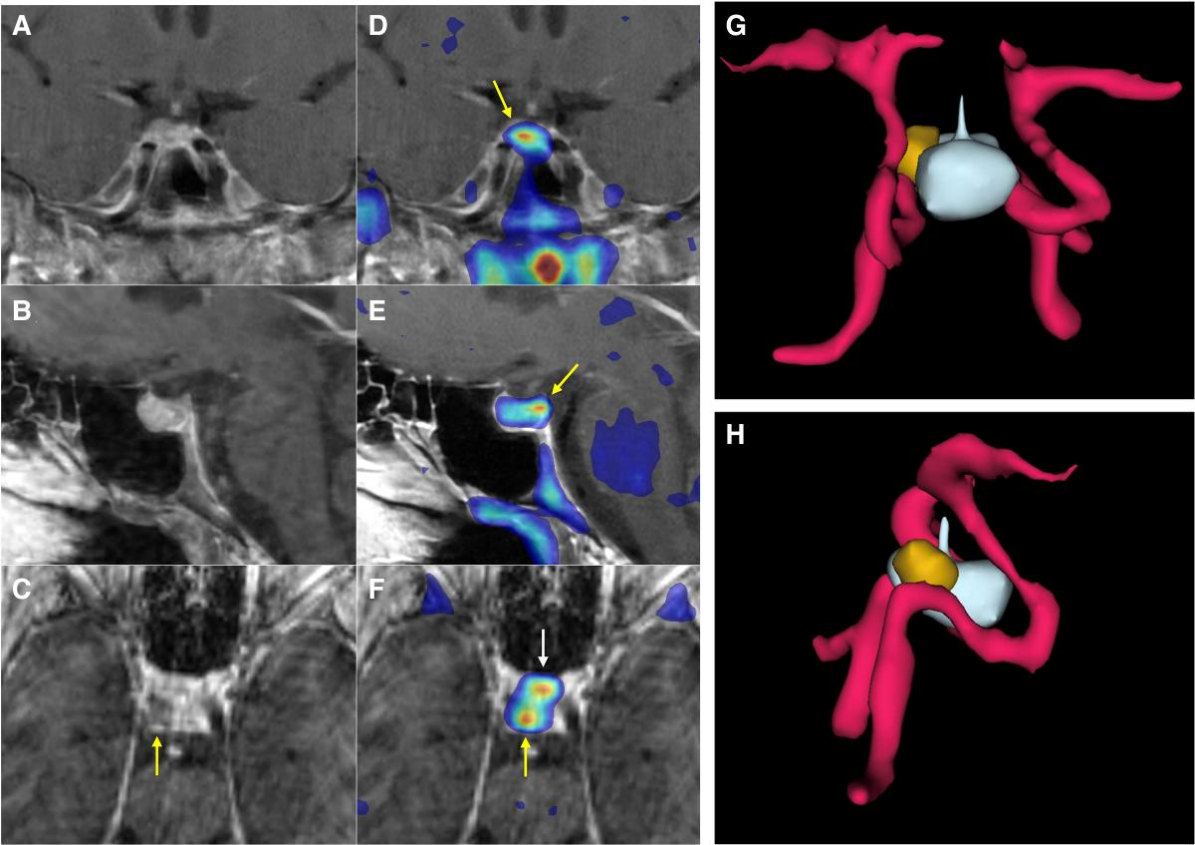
11C-Methionine PET/CT coregistered with volumetric MRI (MET-PET/MR^CR^) following treatment with osilodrostat. A subtle area of reduced gadolinium enhancement can now be appreciated on the right posterosuperior aspect of the gland (A-C). MET-PET/MR^CR^ confirms focal tracer uptake at this site (yellow arrows) and also within normal gland anteriorly (white arrow) (D-F). Three-dimensional reconstruction using CT, MRI, and PET datasets demonstrating the location of the corticotroph microadenoma which was confirmed at subsequent surgery (G-H).
